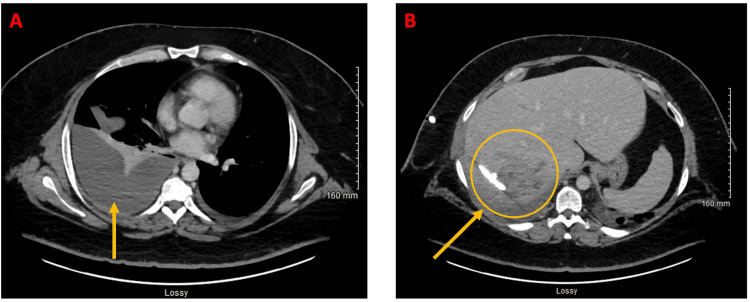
CT of the abdomen/pelvis with contrast on hospital day one (second admission) (A) Axial view of pleural effusion/developing empyema. (B) Axial view of resolving liver abscess with drain.
